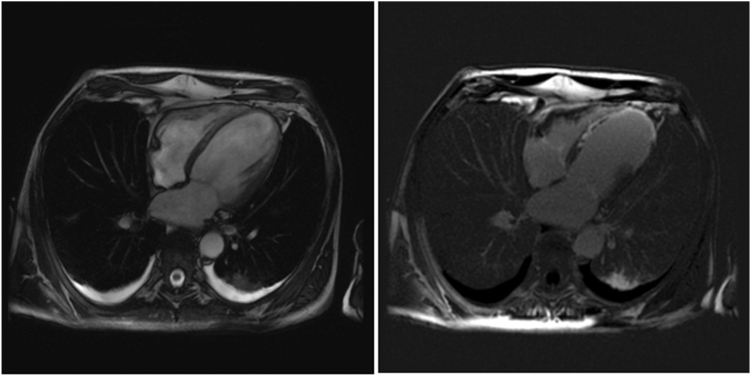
Cardiac magnetic resonance imaging (MRI). Left image: Cine MRI with myocardial edema of the septomedian and septoapical wall. Right image: Myocardial delayed enhancement of the septoapical region.
